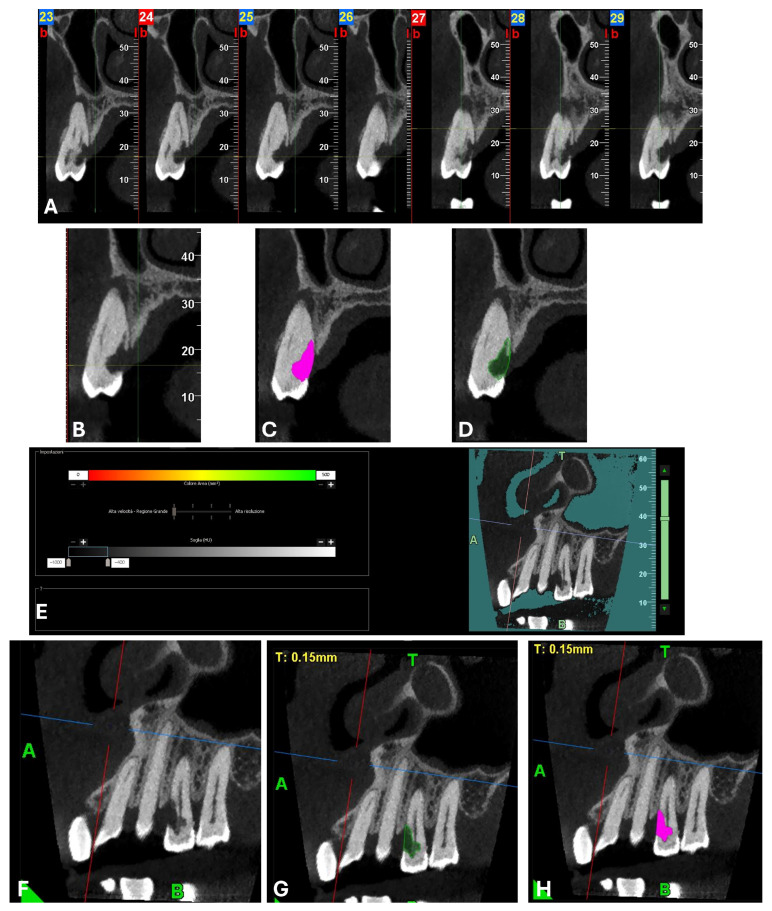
Cross-section and Pano-rex CBCT images of tooth 1.4 and its ECR and EPL. (A,B) Sequential sagittal scrolling allows the evaluation of the lesion in the bucco-lingual direction and its continuity along the root axis; (C,D) Segmentation of Figure 3B using the advanced software functions iRYS^®^ (MyRay, Imola, Italy); (E) Interface of the aforementioned software to obtain segmentation on Pano-rex image. (F) Pano-rex image of tooth 1.4 and its EPL. (G,H) Segmentation of Figure 3F using the advanced software functions. The colored lines crossing the images represent the orthogonal planes (axial, coronal, and sagittal) in the multiplanar reconstruction. Their correct use and orientation allow for an accurate identification of the extent of the lesions in all spatial planes and a detailed analysis of the endodontic anatomy. The green letters are the coordinates of the image: A: anterior, B: bottom, T: Top.
